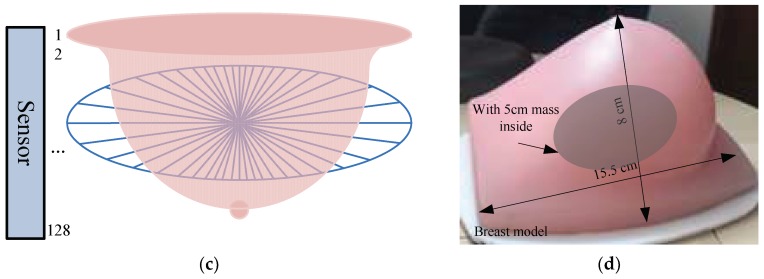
Ultrasound tomography transducer acquisition setup. (a) Placement of breast in the ring; (b) Transducer ring configuration platform; (c) Slice maps of ultrasound tomography imaging; (d) Breast Model.
